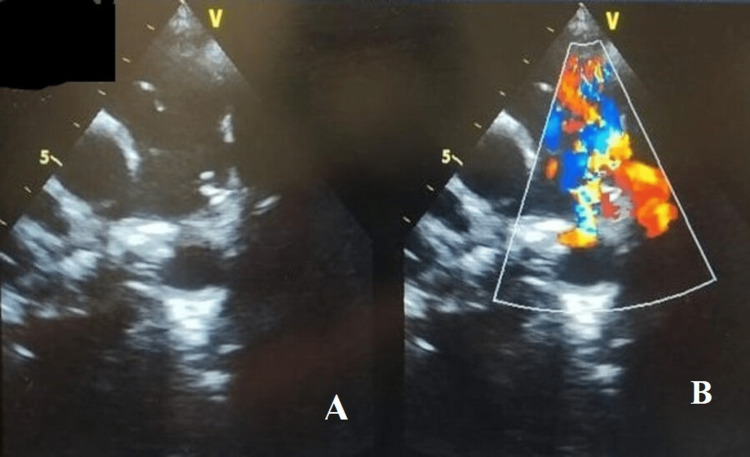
The transthoracic echocardiology (TTE) showed Patent ductus arteriosus (PDA)
